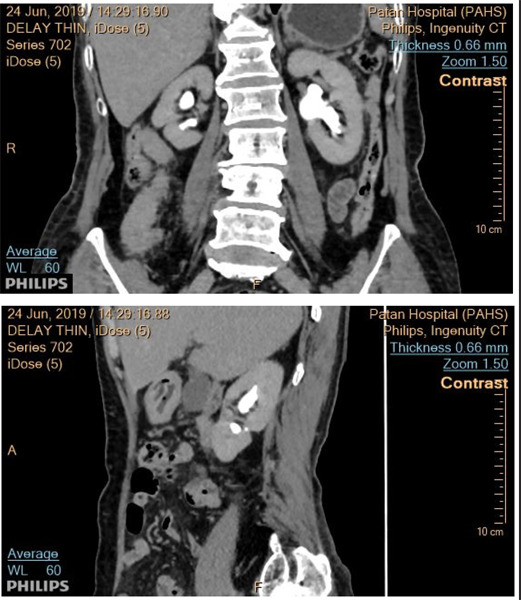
Coronal (A) and right sagittal (B) reconstructed CT urography images in excretory phase showing caudally located fused right supernumerary kidney (arrow) and normal left kidney.
